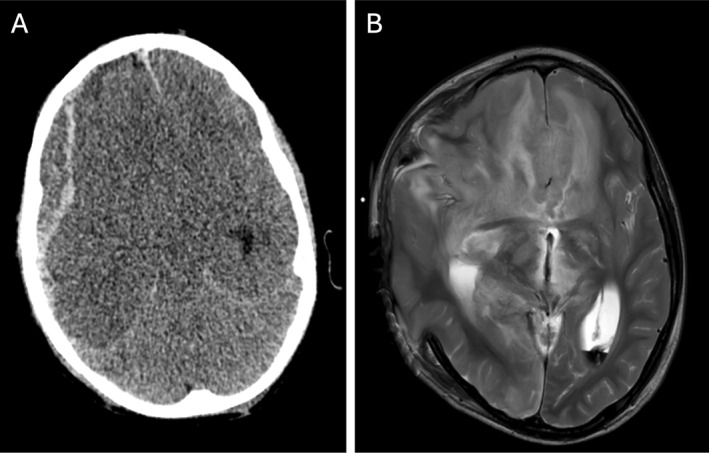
A 6‐year‐old male with significant bilateral retinal hemorrhages, skull fractures, and eventual brain death who presented before the pandemic. (A and B) CT and MRI demonstrate right‐sided subdural hematoma, leftward midline shift, transtentorial/uncal herniation, and infarcts of the entire right cerebral hemisphere and to a lesser degree the left cerebral hemisphere.
